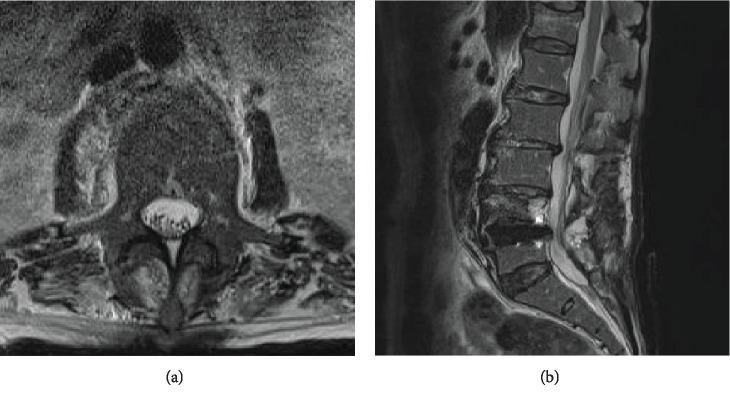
Postoperative MRI imaging after debridement. T2 axial image (a) and sagittal image (b) showing disappearance of mass compressing the cauda equina.
